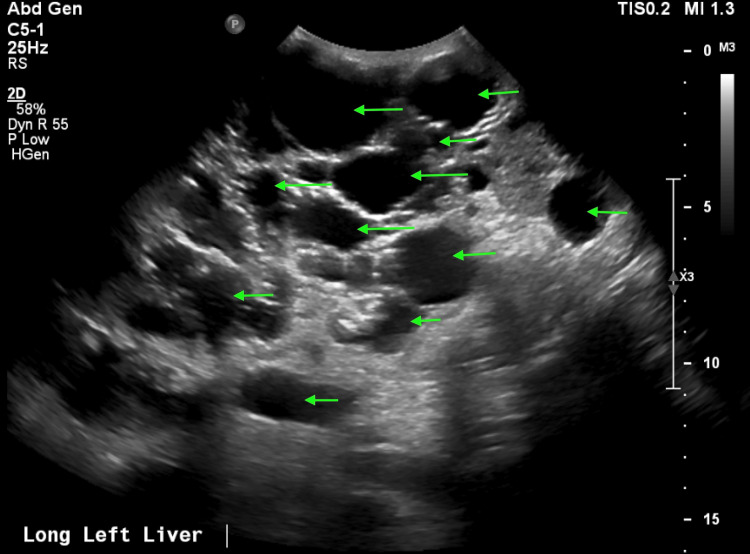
Ultrasound of the liver with several intrahepatic cysts (green arrows)
